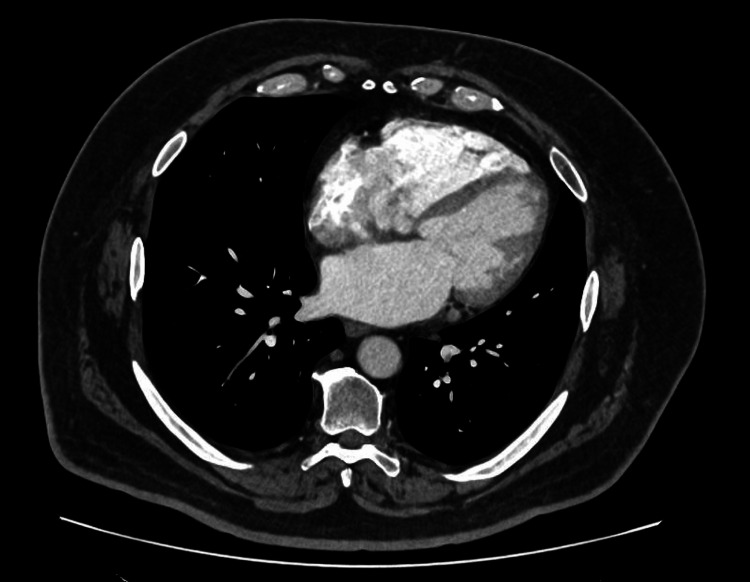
CT Thorax-Cardiac Level Contrast-enhanced CT thorax at cardiac level demonstrating baseline cardiac anatomy prior to immunotherapy initiation.
